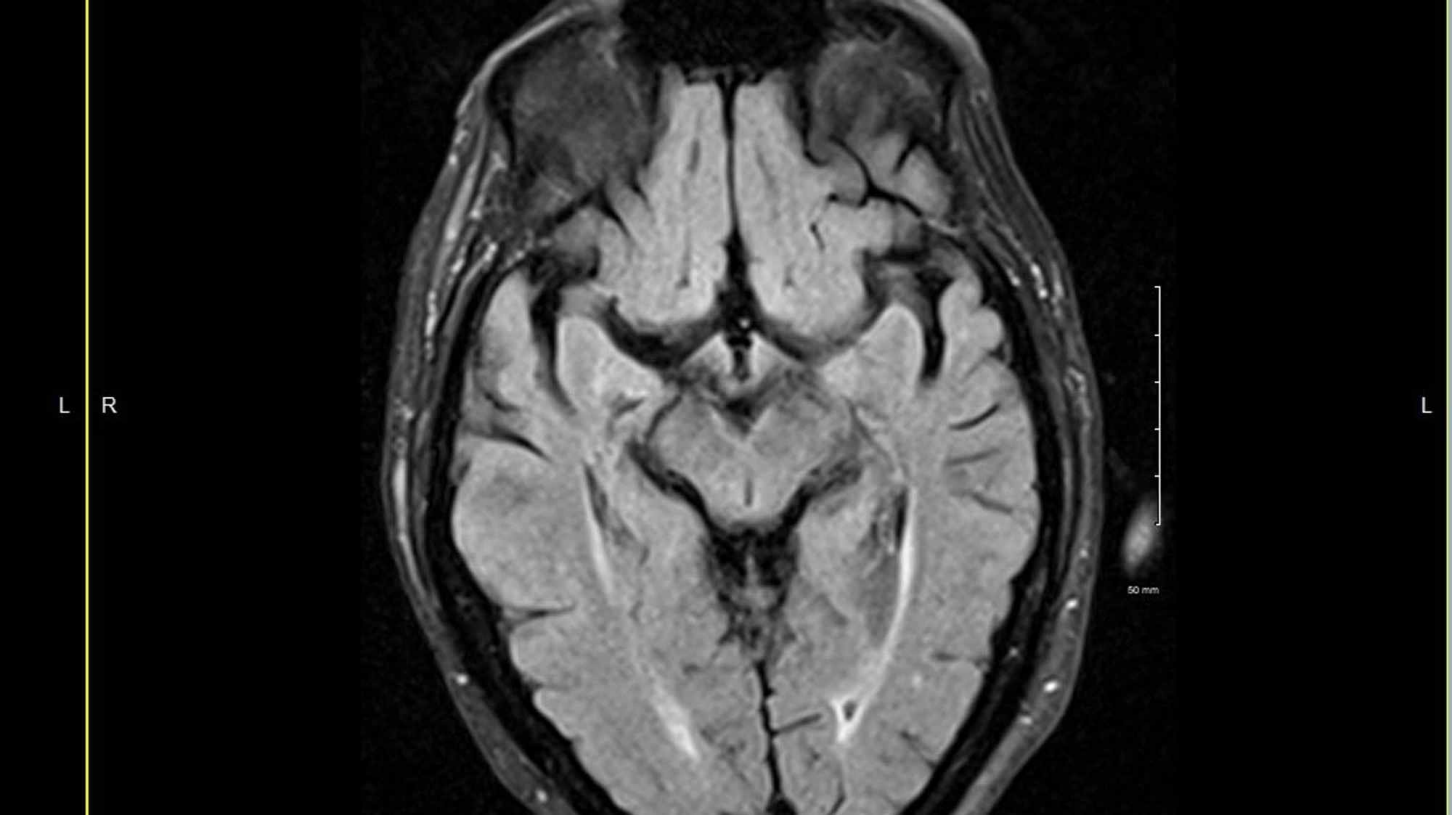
MRI of brain. MRI showing minimal small vessel disease that is not unexpected for age. Otherwise, it was an unremarkable MRI (normal). Electroencephalogram (EEG) was normal as well. No EEG tracings available for publication.
